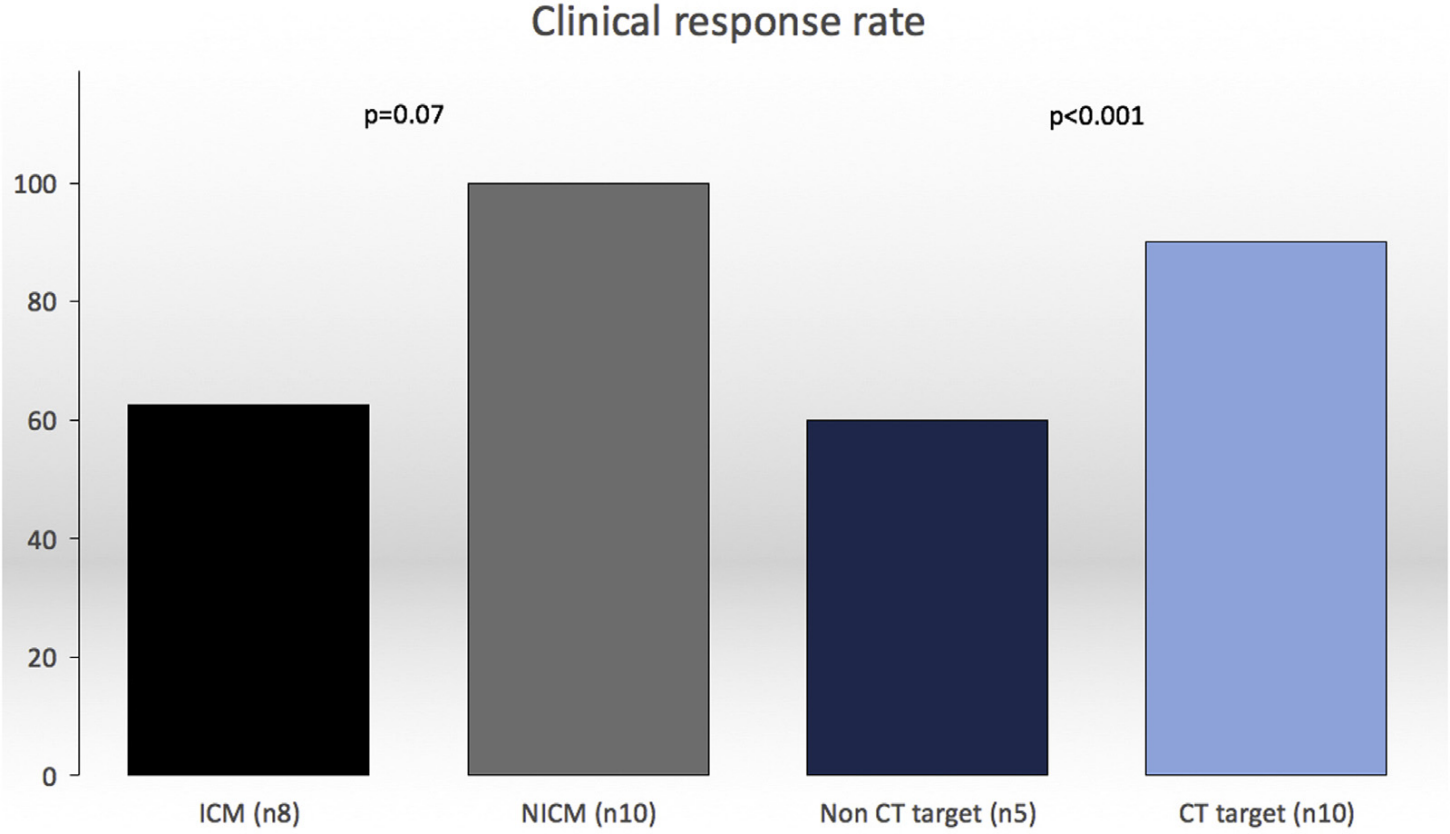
Clinical response rates in CT-SQUEEZ target (n = 10) vs nontarget (n = 5) (*P* < .001) and ICM (n = 8) vs NICM (n = 10) (*P* = .07). CT = computed tomography; ICM = ischemic cardiomyopathy; NICM = nonischemic cardiomyopathy; SQUEEZ = stretch quantifier for endocardial engraved zones.
